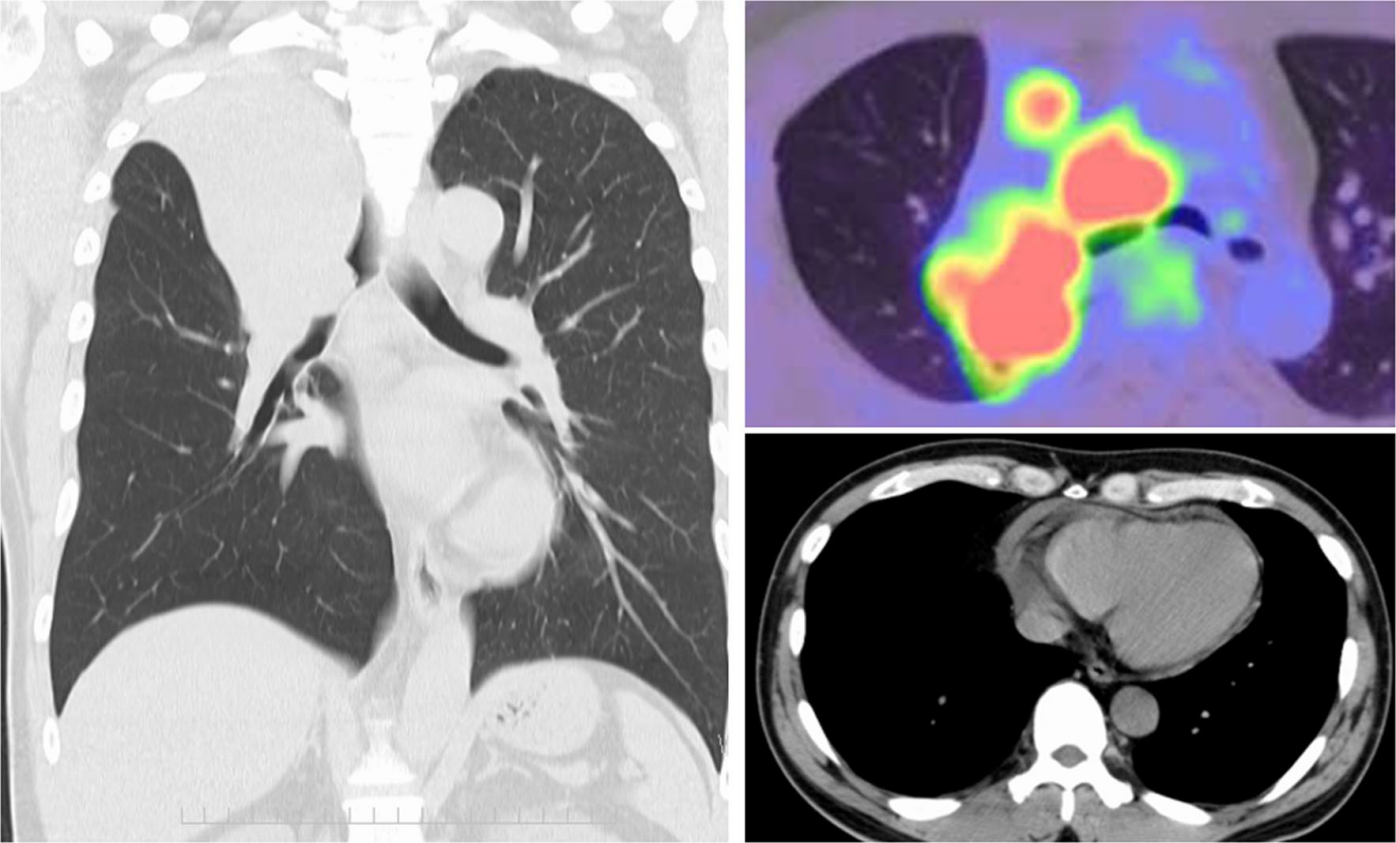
Computed tomography and positron emission tomography at initial diagnosis revealed a large tumor obstructing the right main bronchus (left), enlarged upper and lower paratracheal nodes with strong uptake of fluorodeoxyglucose (right upper), and pericardial effusion (right lower)
